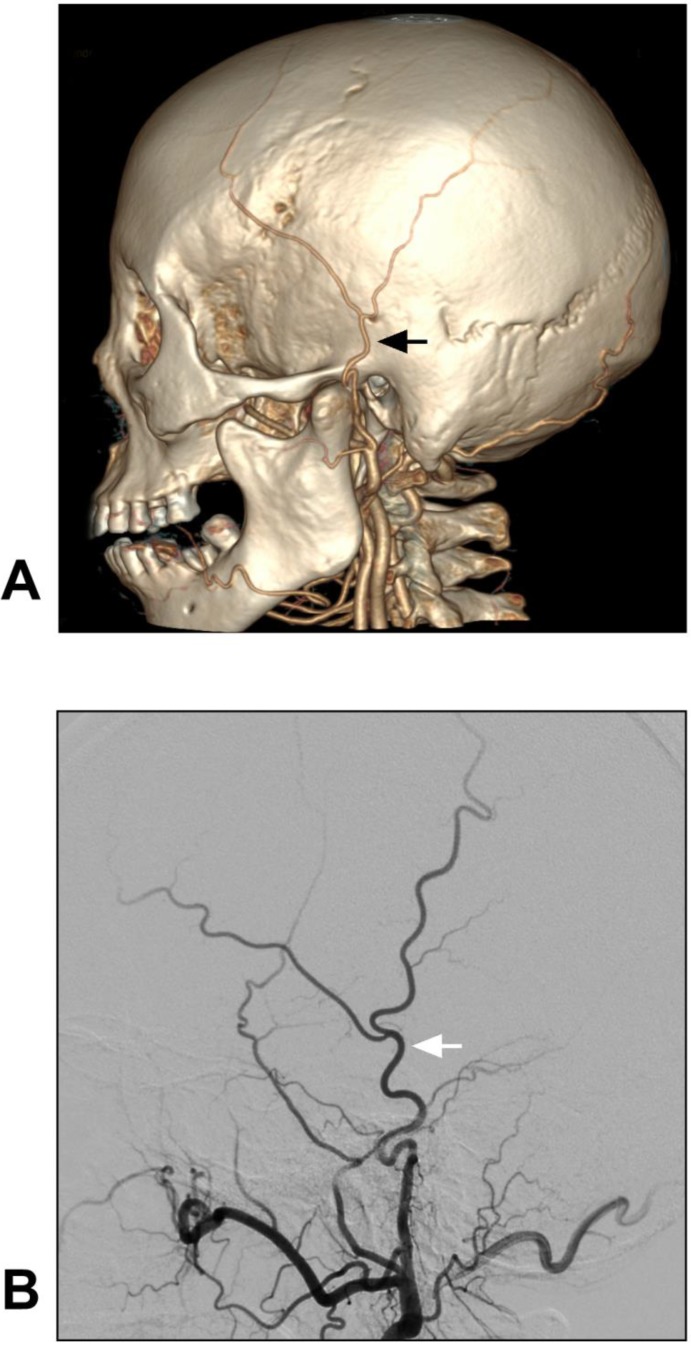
Anatomy of STA on CTA and DSA. A-B: CTA (A) and DSA images of the ECA (B) show the STA courses over the root of the zygoma before it roughly divides into the frontal and parietal branches. The STA is indicated by black and white arrows in A and B respectively. These CTA and DSA images were obtained from different patients. Abbreviations: CTA: computed tomography angiography; DSA: digital subtraction angiography; ECA: external carotid artery; STA: superficial temporal artery.
